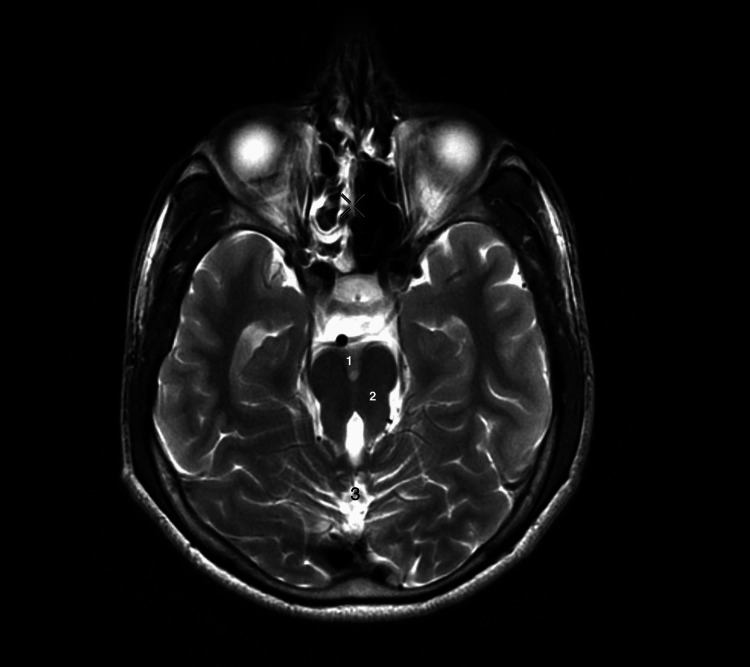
T2-weighted brain MRI in axial view of the sibling showing deep interpeduncular fossa, elongated bilateral superior cerebellar peduncle, and hypoplastic vermis consistent with Joubert syndrome
